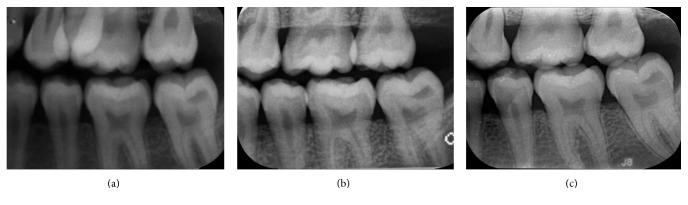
Bitewing radiographs of the PEIR-affected tooth (permanent mandibular left second molar) when the patient was (a) 13 years, 4 months old, (b) 15 years, 3 months old, and (c) 17 years, 4 months old. The PEIR has no connection to the enamel or the pulp of the affected tooth.
